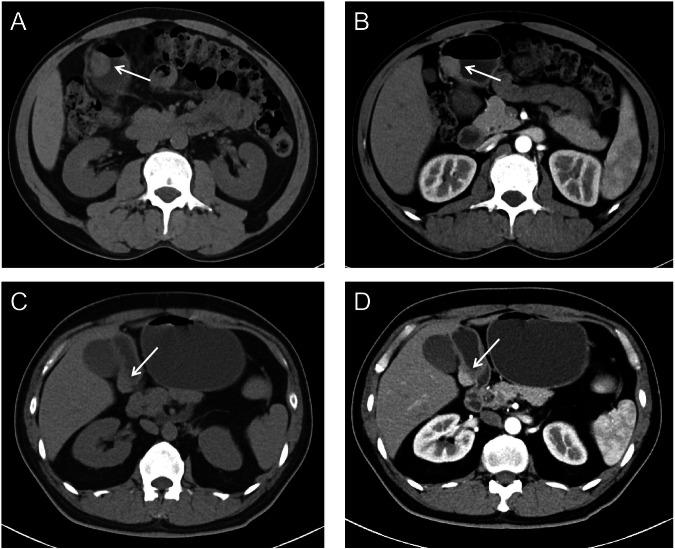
Representative multiphase CT images of gastric ectopic pancreas (GEP) and gastric stromal tumors (GIST, white arrows). A non-contrast phase of GEP, B arterial phase of GEP, C non-contrast phase of GIST, D arterial phase of GIST
